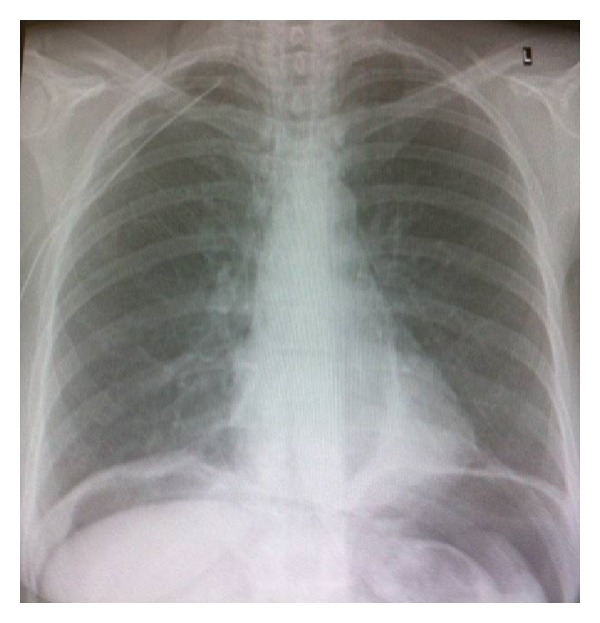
Chest X-ray after the insertion of a right-sided chest tube. Pneumothorax has completely resolved. Existence of Intraperitoneal free air.
